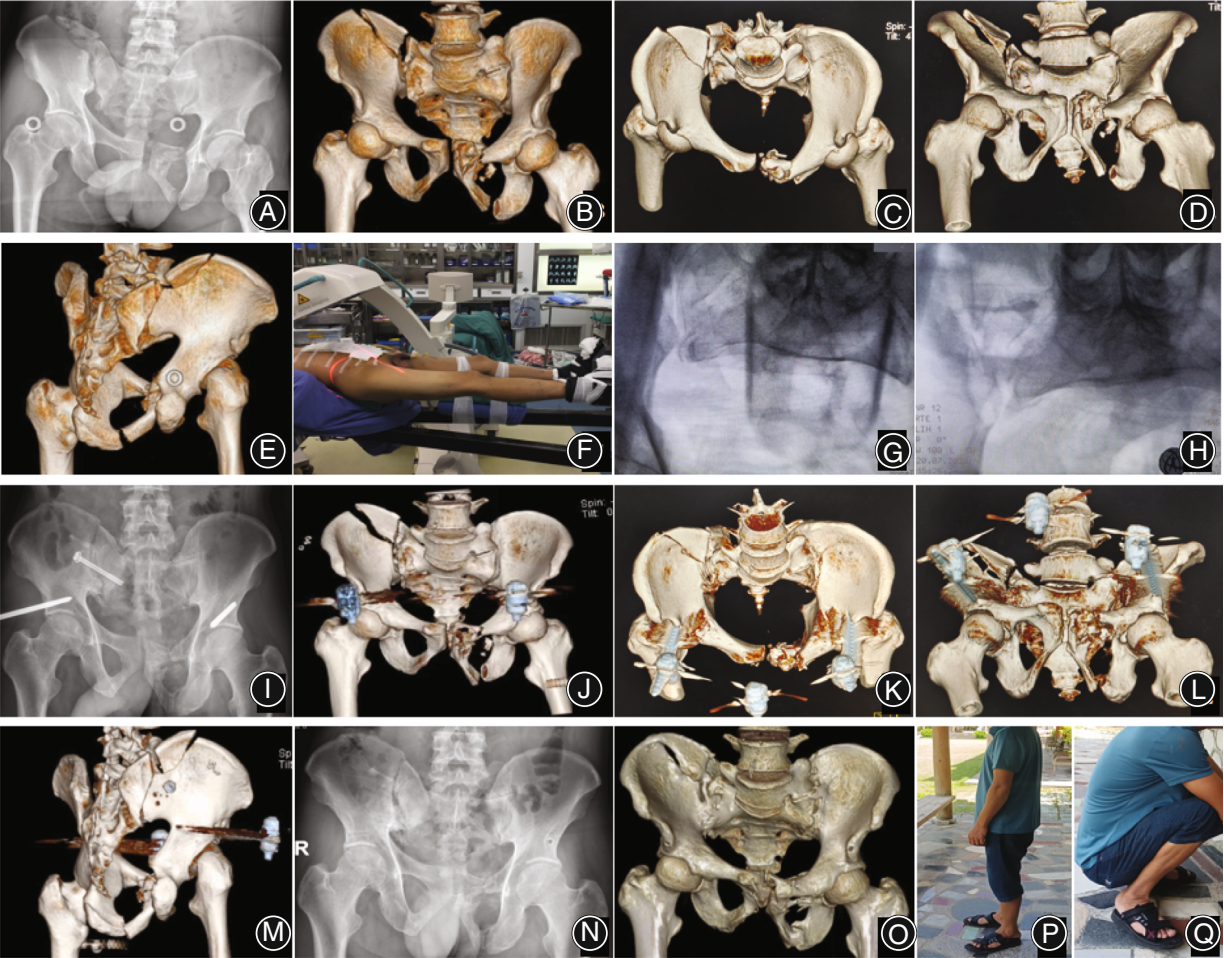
A 42‐year‐old man with pelvic ring injuries (Case 2) caused by a heavy crush injury. (A–E) Preoperative plain x‐ray radiographs and three‐dimensional computed tomography images showing a right iliac fracture with sacroiliac joint dislocation and left pubic ramus fractures (F–H) The countertraction closed reduction technique to reduce the fractures. (I–M) Two days postoperative plain X‐ray radiograph and three‐dimensional computed tomography images showing good reduction of the pelvic ring. (N, O) Plain radiographs and three‐dimensional computed tomographic images three‐months postoperatively showing healing of the pelvic fracture and the external fixator was removed. (P, Q) Follow‐up 2 years after surgery showed excellent function.
